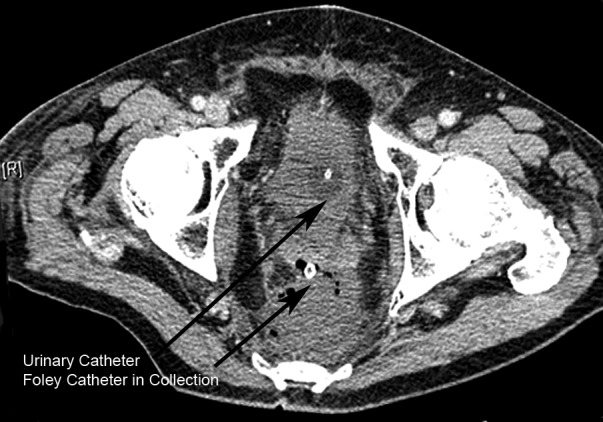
Computed tomography demonstrating pelvic fluid and gas collection incompletely drained by a rectal foley catheter
